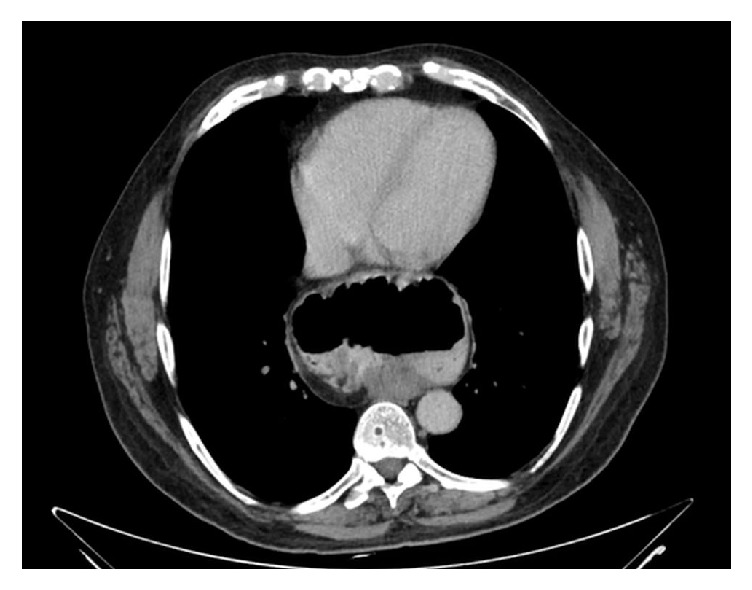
CT scan showing huge hiatal hernia and upside-down stomach.
